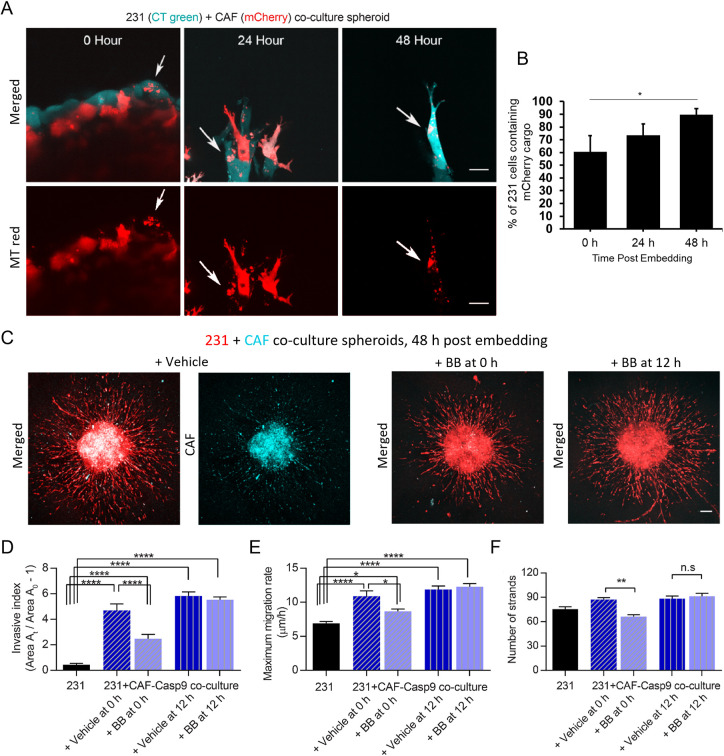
Initial presence of CAFs during migration enhances migratory capabilities. (A) Co-culture spheroids of CAF01-mCherry and CellTracker-labelled MDA-MB-231 cells (CT Green) at 0, 24 and 48 h post embedding (white arrows indicate transferred cargo from CAFs to cancer cells). Scale bars: 20 µm. (B) The number of MDA-MB-231 cells containing mCherry signal increases each day, with the increase being statistically significant between 0 h and 48 h (*n*=59, 38, 34 cells across 7, 7 and 11 independent images, respectively). (C) Representative images of co-culture spheroids of CellTracker-labeled MDA-MB-231 (red) and CAF01-Casp9-GFP (cyan) cells 48 h post embedding. Spheroids were treated with B/B homodimerizer to induce caspase-9 expression and apoptosis in CAFs at 0 h or 12 h post embedding or with vehicle control. Scale bar: 50 µm. (D,E) Induction of caspase-9 with the B/B homodimerizer at 12 h post embedding to kill the CAF01-Casp9 cells in the co-culture spheroid minimally affects the invasive index (D; *n*=17, 8, 17, 16 and 21 spheroids, respectively) and maximum migration rate (E; *n*=16, 10, 14, 16, and 19 spheroids, respectively) measured at 48 h post embedding, whereas treating the co-culture spheroid with B/B immediately (0 h) post embedding decreases the CAF-promoted cancer cell migration. (F) Induction of caspase-9 at 12 h post embedding to kill the CAF01-Casp9 cells in the co-culture spheroid minimally affects the number of strand protrusions (*n*=17, 10, 14, 16 and 19 spheroids, respectively) measured at 48 h post embedding, whereas treating the co-culture spheroid with B/B immediately (0 h) post embedding decreases the CAF-promoted increase in number of strand protrusions. All data pooled from a minimum of three independent experiments. Error bars are mean±s.e.m. n.s., not significant (*P*>0.05); P*<0.05; *P*<0.01; *P*<0.0001 [one-way ANOVA followed by Sidak's (D–F) or Tukey's (B) multiple comparison testing].
